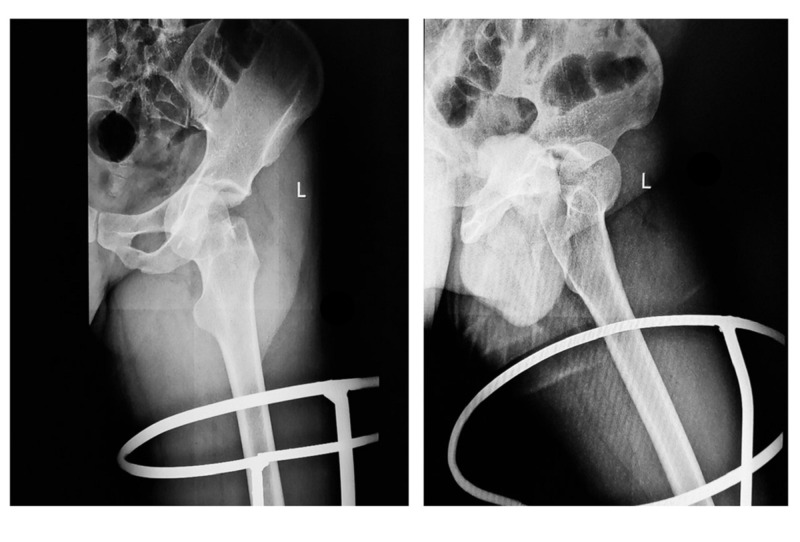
AP and lateral X-ray Anteroposterior (AP) and lateral X-ray of left hip showing pubic-type anterior dislocation of the left hip
